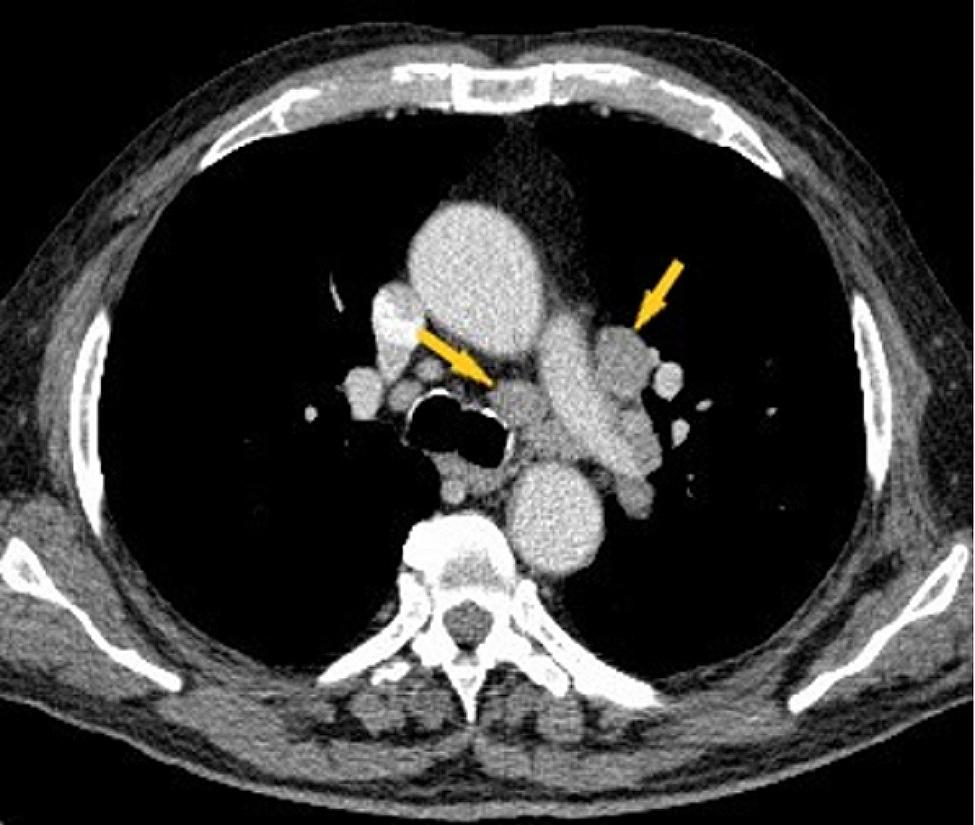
Chest CT examination, venous time, axial section, mediastinal window: mediastinal adenopathy involving the left branch of the pulmonary artery (yellow arrows)
